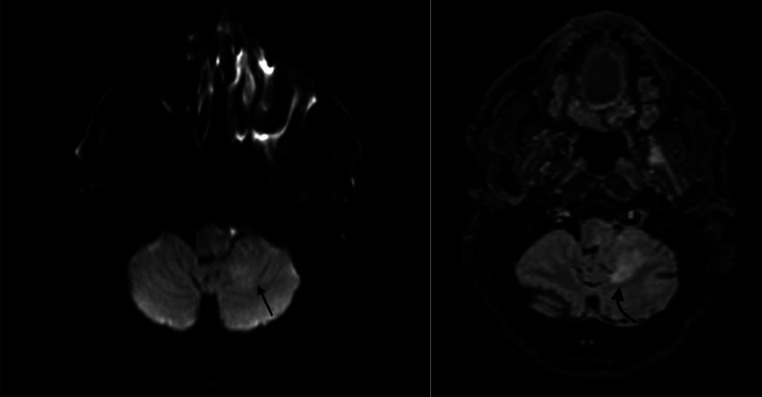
Axial FLAIR weighted sequence/ Diffusion-weighted sequence revealed ischemic signal anomaly at the left bulbo-cerebellar level (Curved arrow), hyperintense in FLAIR (A), showing mild diffusion hyperintensity, with high apparent diffusion coefficient (ADC), well involving the left vagus et glossopharyngeal nerves nucleus (straight arrow), consisting in venous infarcts (B).
